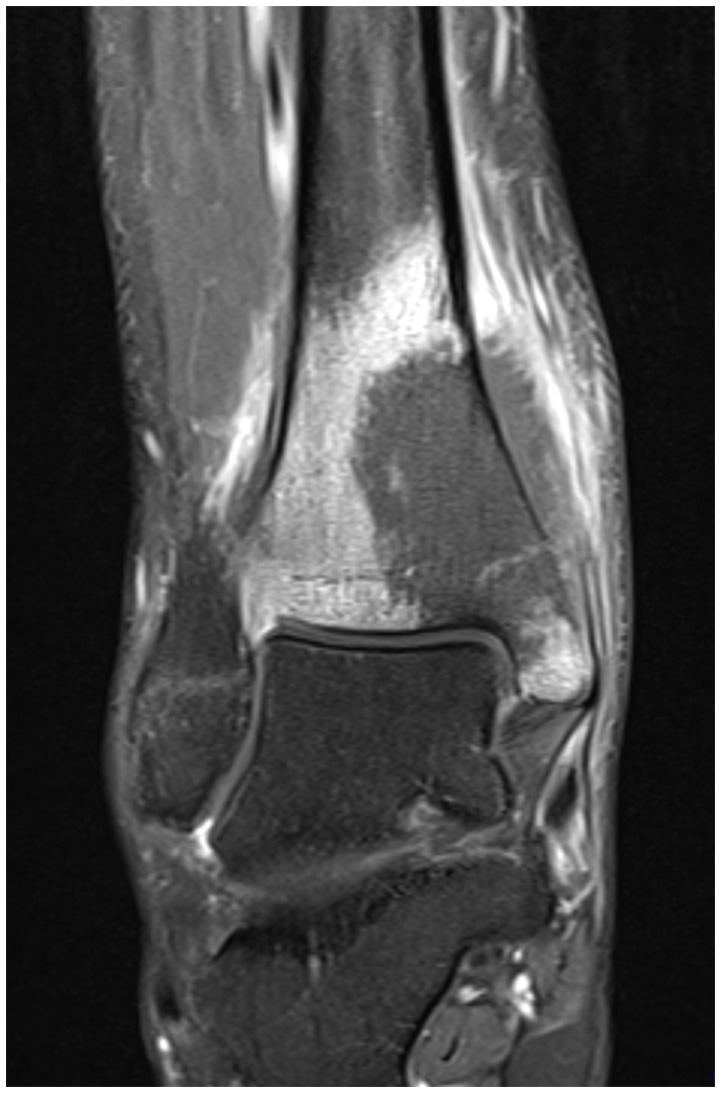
MRI, coronal section of right ankle, T1 weighted image.
